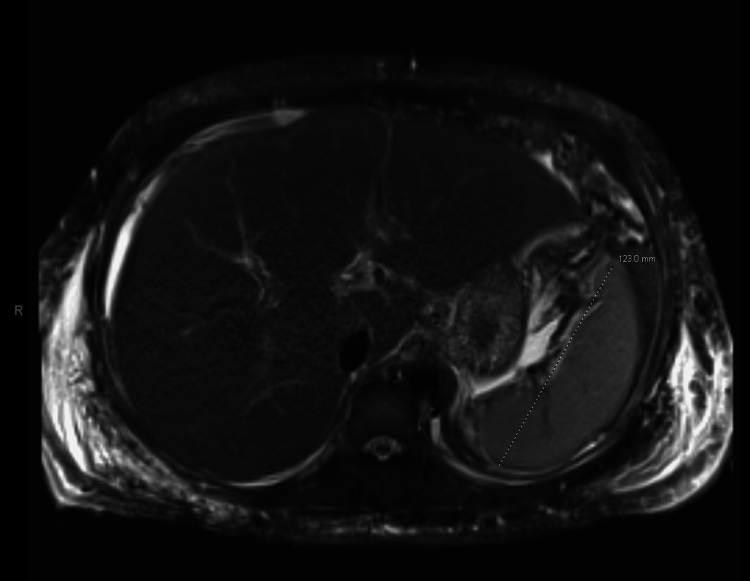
MRI abdomen on day 8 of hepatic manifestations Imaging shows severe hepatic steatosis, moderate volume ascites, possible cirrhosis, and a normal spleen size of 12.3 cm. MRI: magnetic resonance imaging
